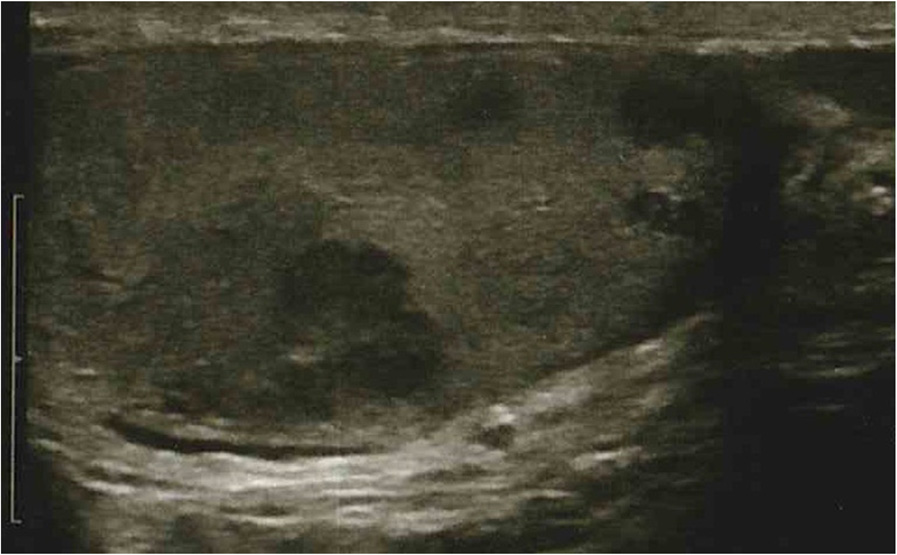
Scrotal ultrasound. Structural changes of the entire right testis
